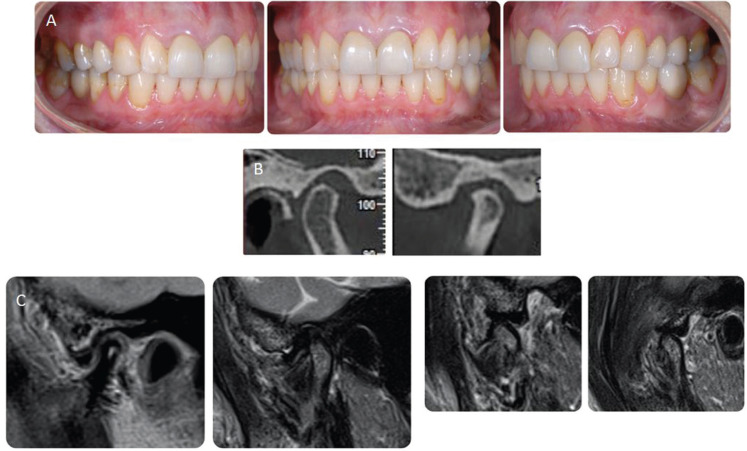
20 years follow up records. A) Intraoral views 20 years after the end of treatment showing good stability of occlusion. B) Serial tomography reconstruction by CBCT 20 years after the treatment showing reshaping of condyles. C) MRI 20 years treatment closed and open mouth shows anterior disc displacement bilaterally.
